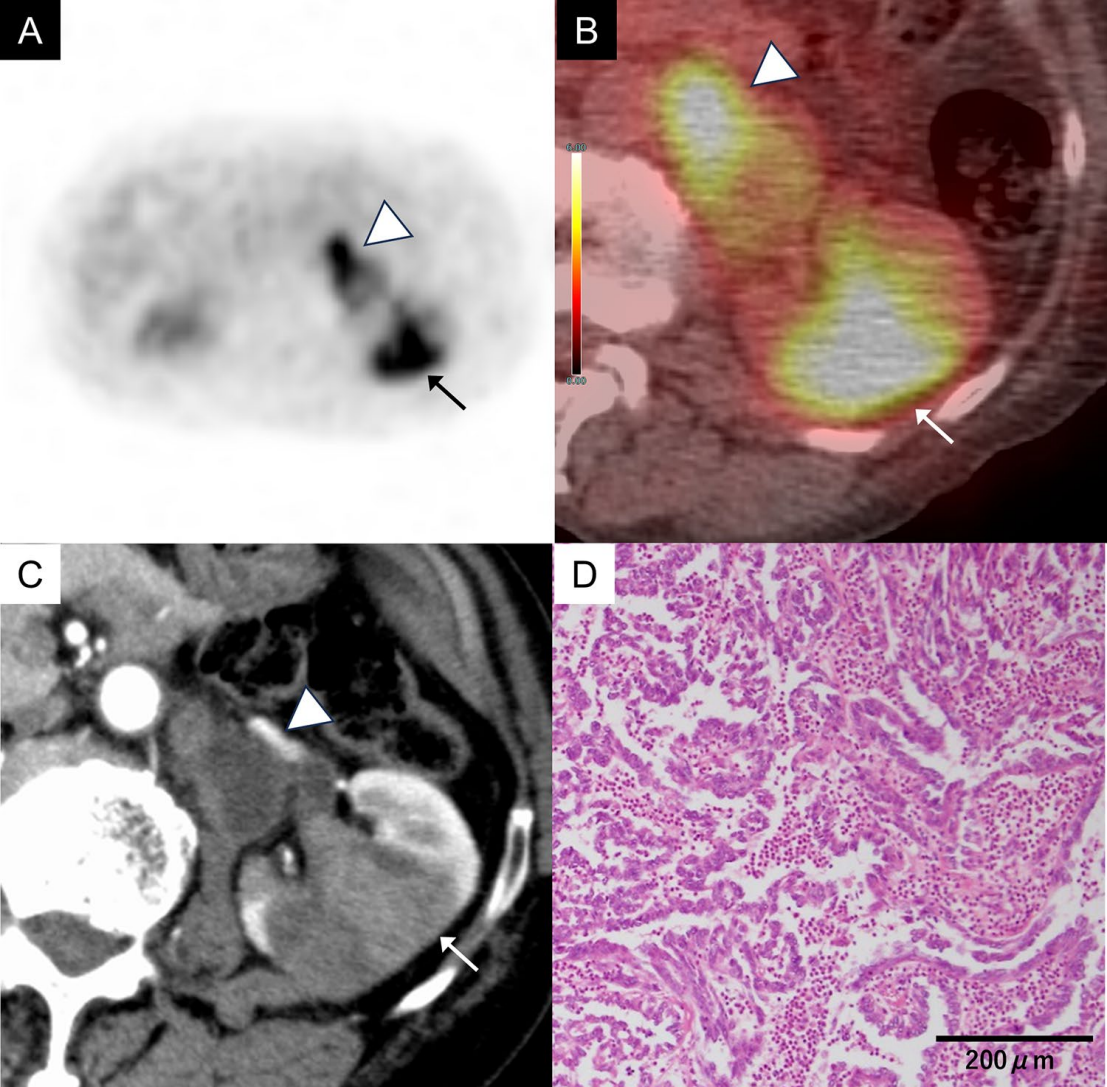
A 77-year-old woman with a collecting duct carcinoma of the left kidney. A Axial FDG-PET image (grayscale) shows moderate FDG uptake in left kidney (arrow) and hilar lymph nodes of the left kidney (arrowhead). B Axial FDG-PET/CT image shows moderate FDG uptake in a mass of the left kidney (arrow), showing an SUVmax 7.8, while normal renal cortex shows an SUVmax 2.2. FDG uptake is also increased in hilar lymph nodes of the left kidney (arrowhead), suggesting a metastasis of the tumor of the left kidney. C Axial contrast-enhanced CT in the corticomedullary phase shows a poor enhancement in the mass (arrow) and the enlarged hilar lymph nodes of the left kidney (arrowhead). D Tumor cells are forming papillary and glandular structures
